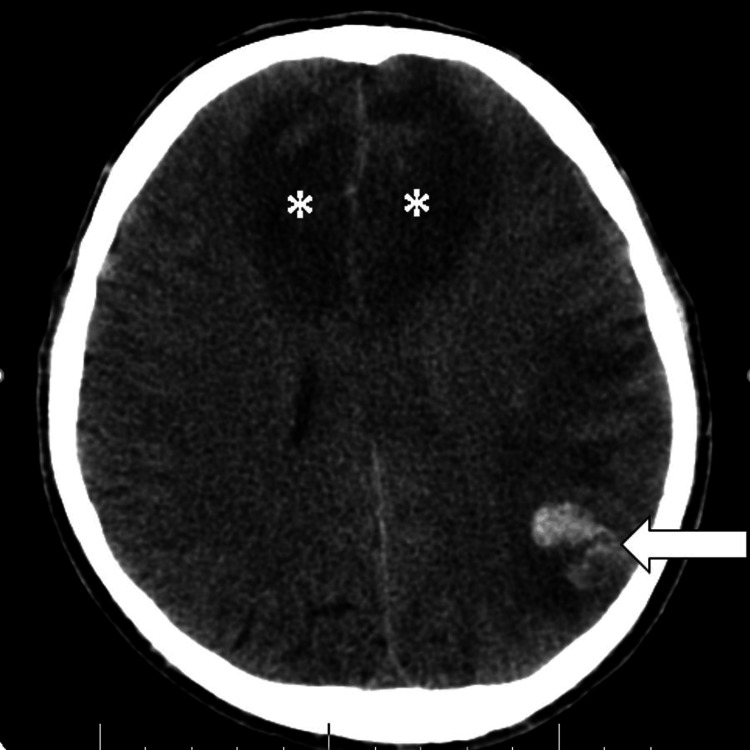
Axial non-enhanced CT scan of the brain. Left posterior parietal intraparenchymal hemorrhage and surrounding edema (white arrow), with bilateral frontal cortico-subcortical hypodensities consistent with cytotoxic edema (asterisks) CT: computed tomography
